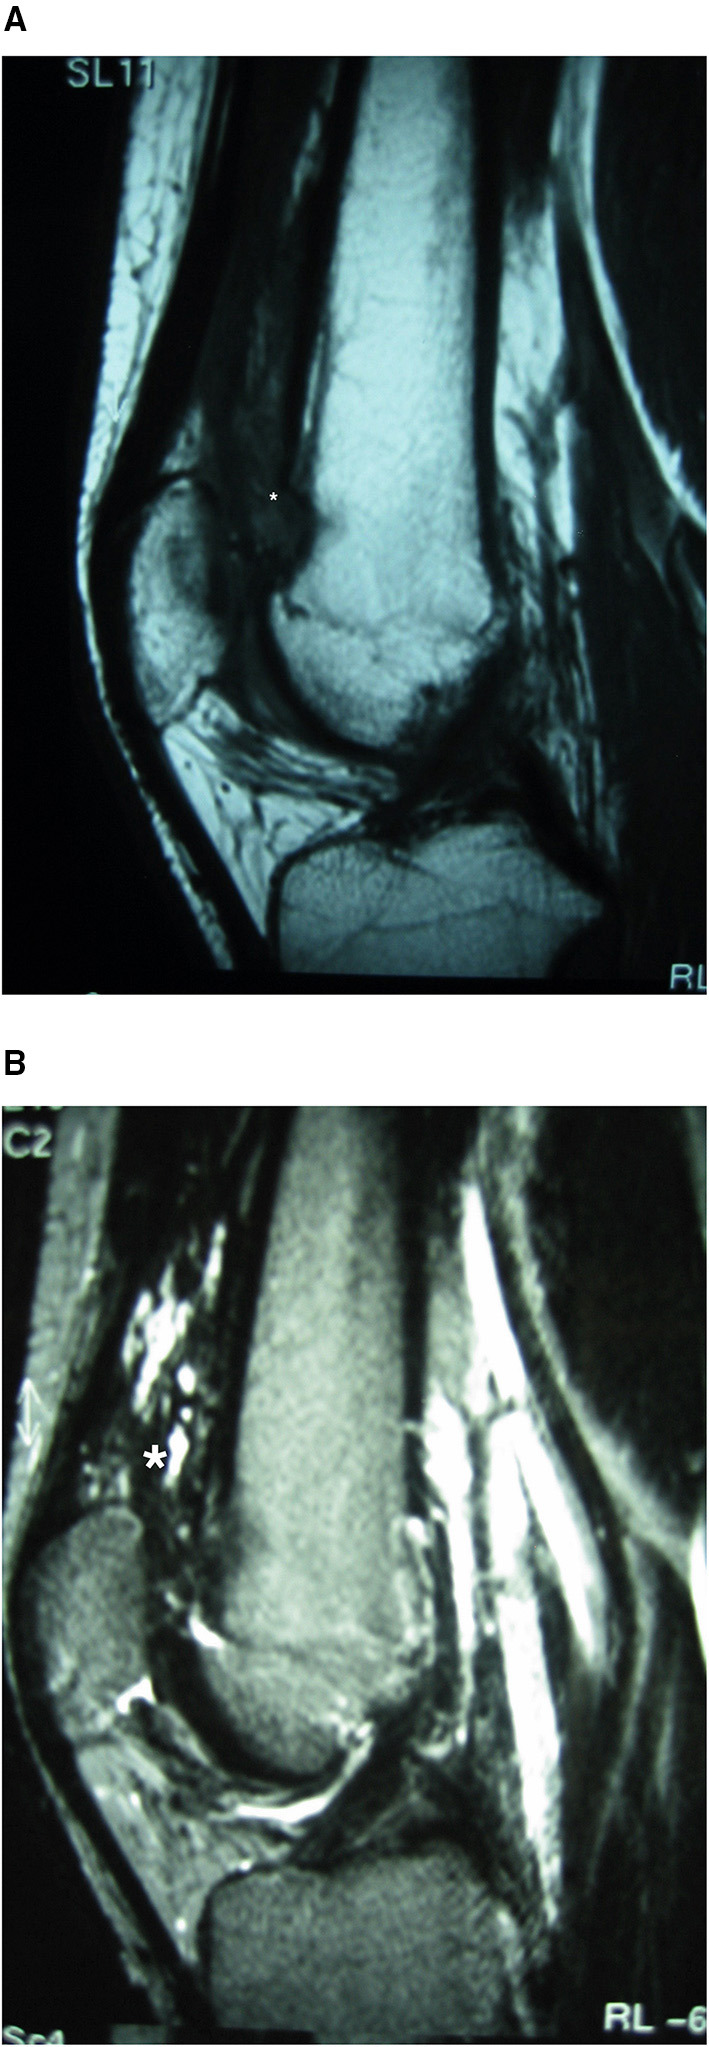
Case 1: suprapatellar MRI tumor recurrence. MRI showing recurrence of the lesion. A heterogeneous hyperintense lesion is observed on sagittal T2-weighted imaging (B); it contains fibrous septa and is hypo-/isointense to muscles on the T1 sequence (A), with diffuse involvement of the sub-quadriceps synovial sac and tendon. *Location of the Synovial Haemangioma tumor in the knee.
